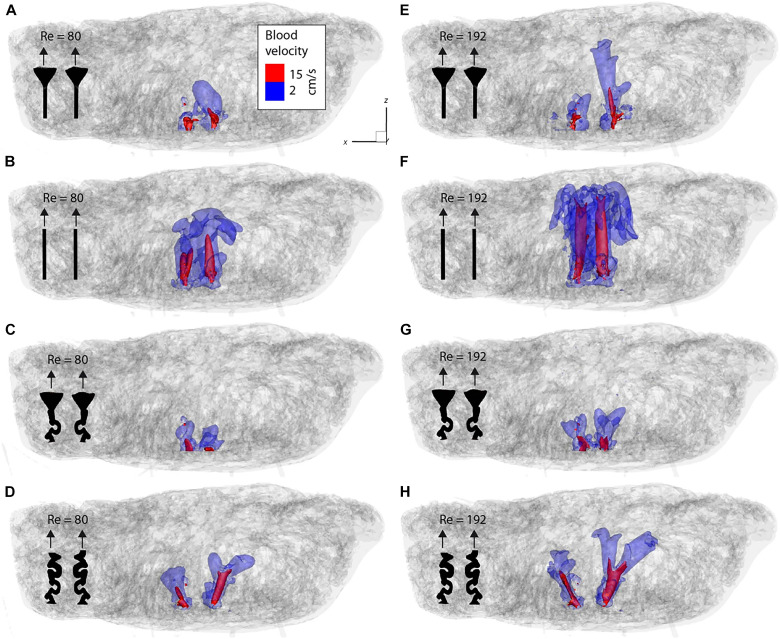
Isovolumes of the fluid velocity. (A to H) Overview of all eight cases measured with velocity-encoded MRI. The gray geometry is the segmented volume from MRI. The colored surfaces indicate the isovolumes where the blood velocity in the placenta would exceed 2 cm/s (blue) and 15 cm/s (red). The black pictograms indicate the artery geometry.
